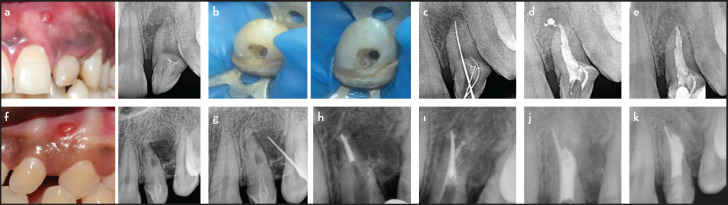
Type II DI cases: Case 1 depicts Type IIA root canal configuration (a) and its management (b-d) with 1 year follow up radiograph showing resorbed sealer (e); Case 2 depicts Type IIB root canal configuration (f, g) and its management using sectional obturation apical to the resorptive defect (h) and MTA obturation in remaining root canal space including resorptive defect (ı) post obturation and post- op radiograph (j, k)
